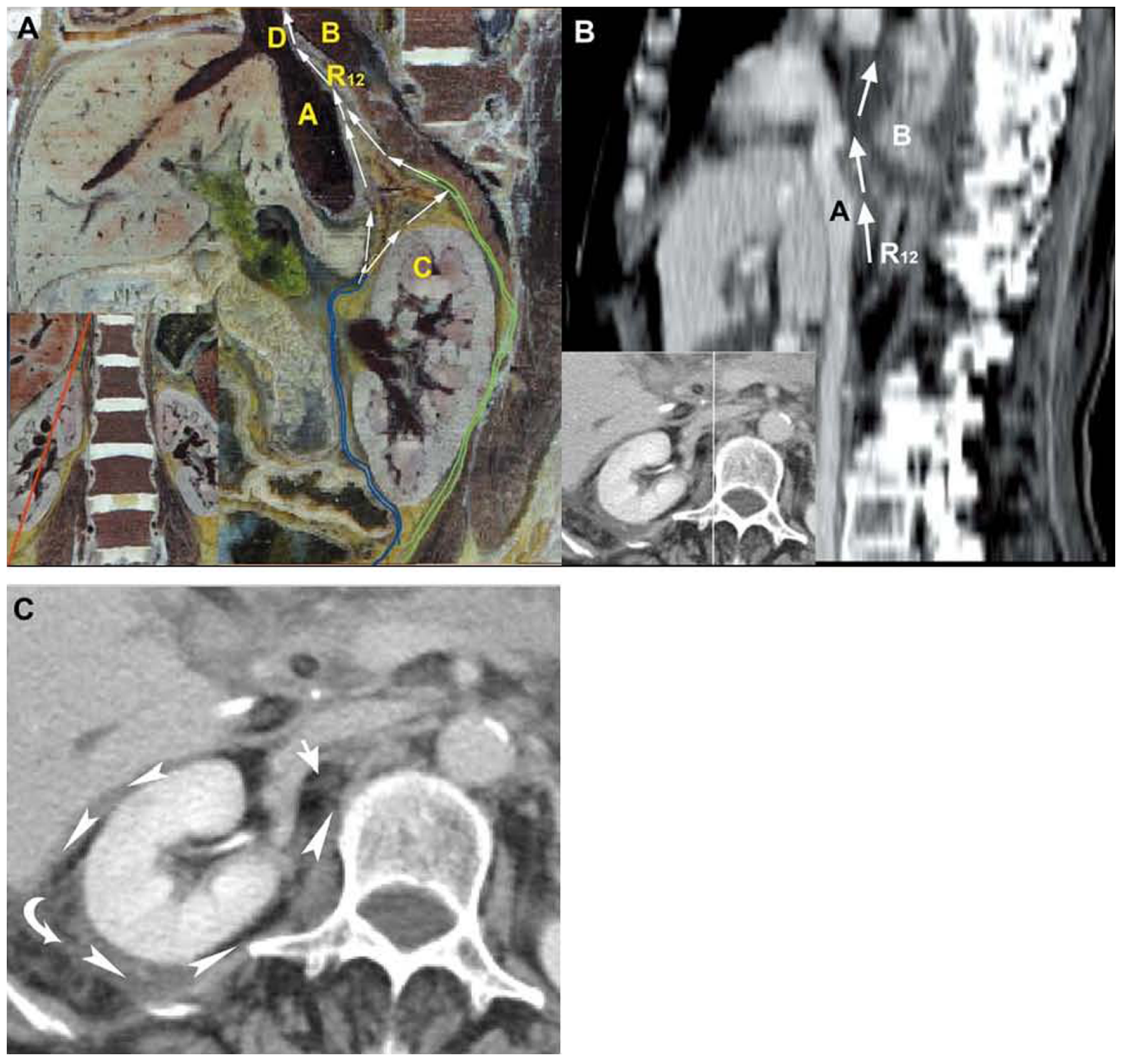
Presentation of the pathways into the right mediastinum via the inferior vena cava hiatus. (A) The oblique sagittal plane shows the potential, cranial Route 12 (long arrow) from the retromesenteric plane (blue lines) and retrorenal plane (green lines) to the bare area of the liver, and further into the right mediastinum via the inferior vena cava hiatus from the medial inferior view on CVH2. (B, C) A 72-year-old woman with recurrent acute pancreatitis. Sagittal plane shows Route 12, in which the fluid drains from the bare area of the liver to the right mediastinum across the inferior vena cava hiatus (long arrow). The peripancreatic fluid in the mediastinum further develops to the pleural effusion and the right lower lobar lung consolidation. The axial CT image depicts the pancreatic fluid flows from the retromesenteric plane to the right retrorenal plane through the right fascial trifurcation (arrowheads) and the bridging septa (short arrow). A: inferior vena cava, B: right lung, C: right kidney, D: inferior vena cava hiatus.
